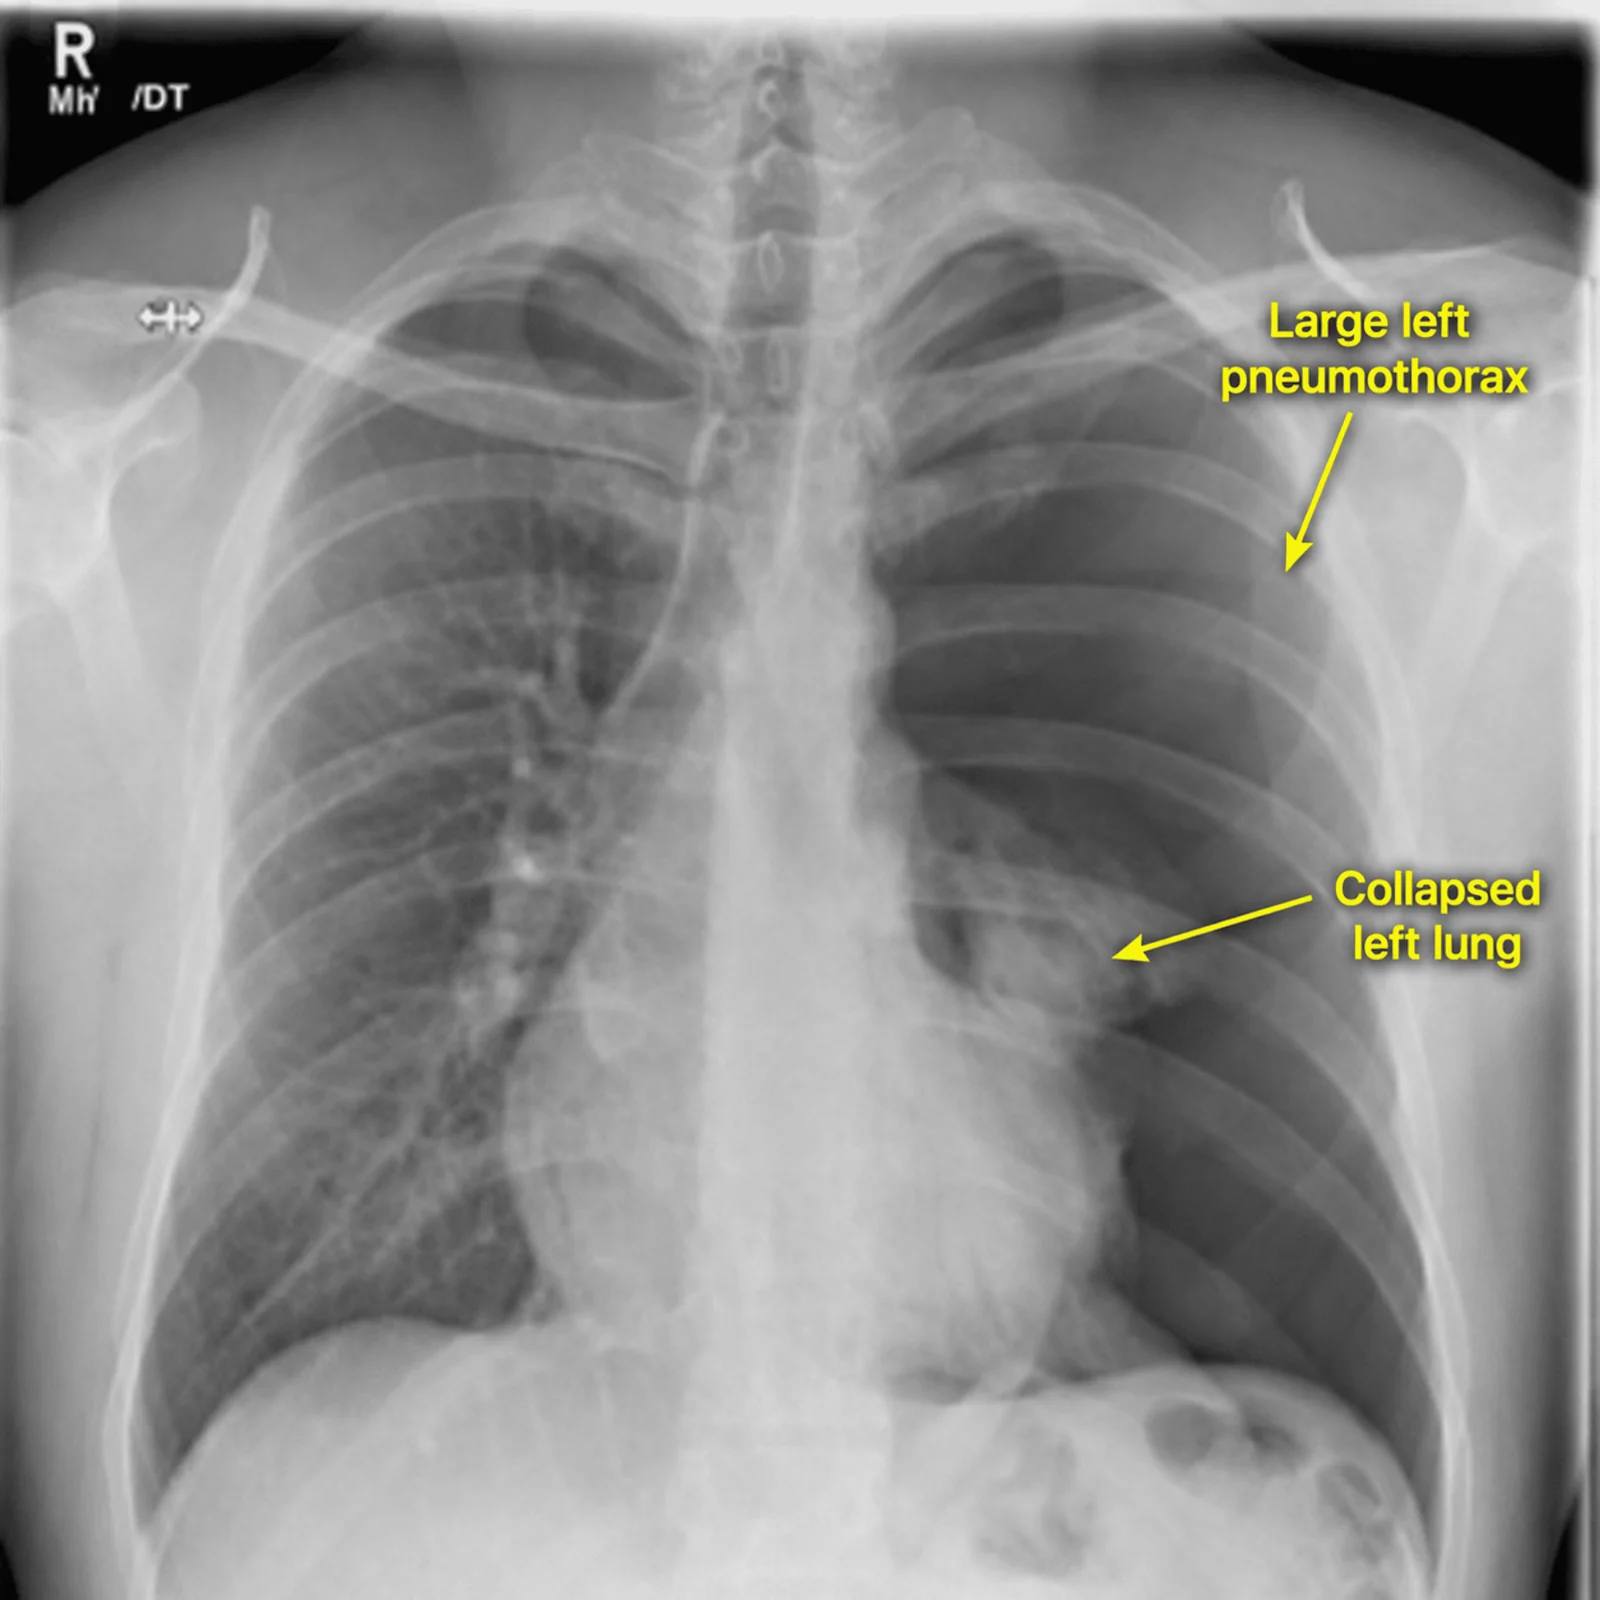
Initial chest radiograph: large left-sided pneumothorax with near-complete collapse of the left lung and subtle mediastinal shift, concerning for evolving tension physiology The annotations identify the visceral pleural line, extensive pleural air, collapsed left lung, and mediastinal displacement.
